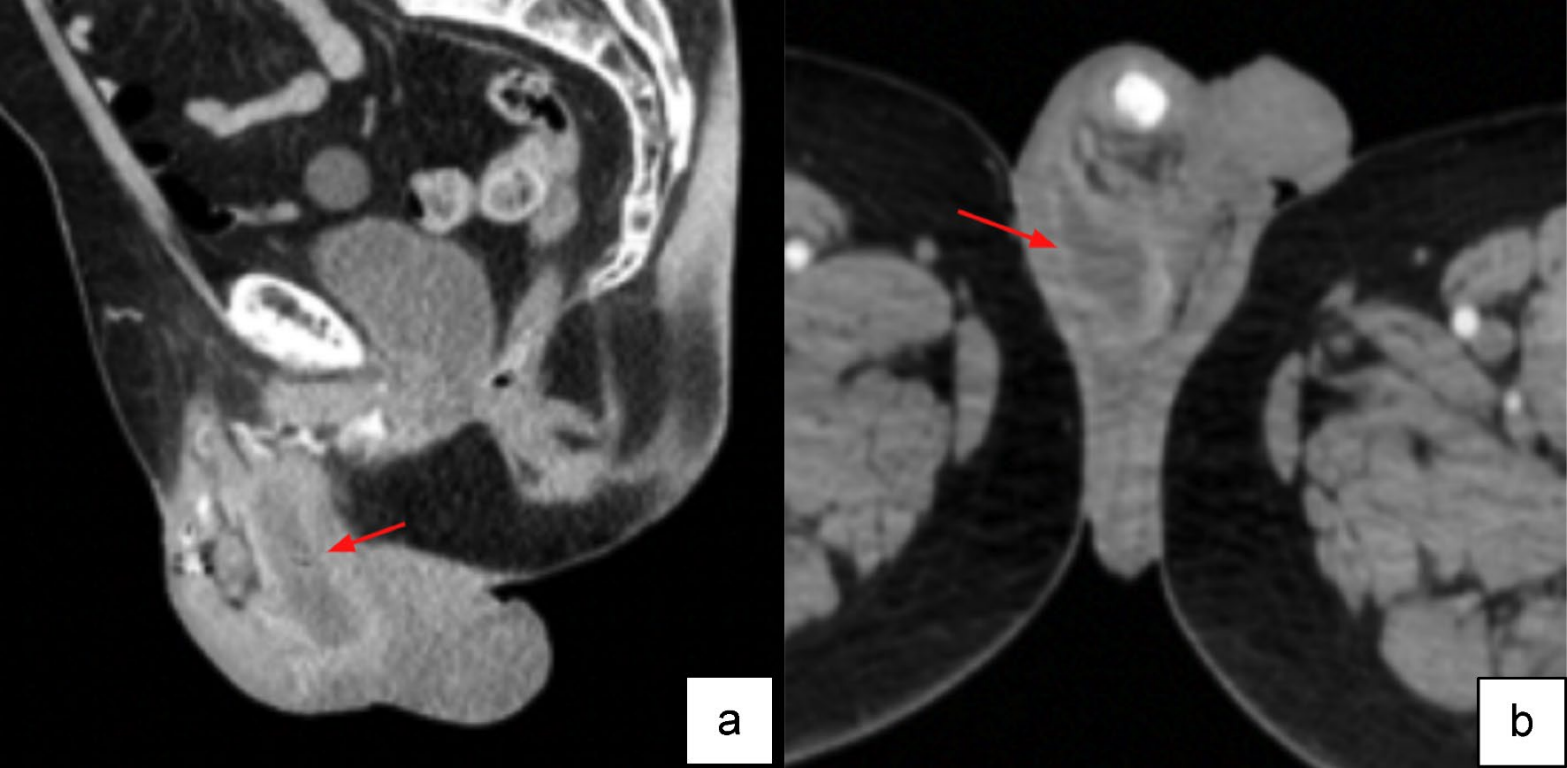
Sagittal (a) and axial (b) CT images with IV contrast show an intrascrotal peripherally enhancing fluid collection (red arrows), consistent with an abscess
